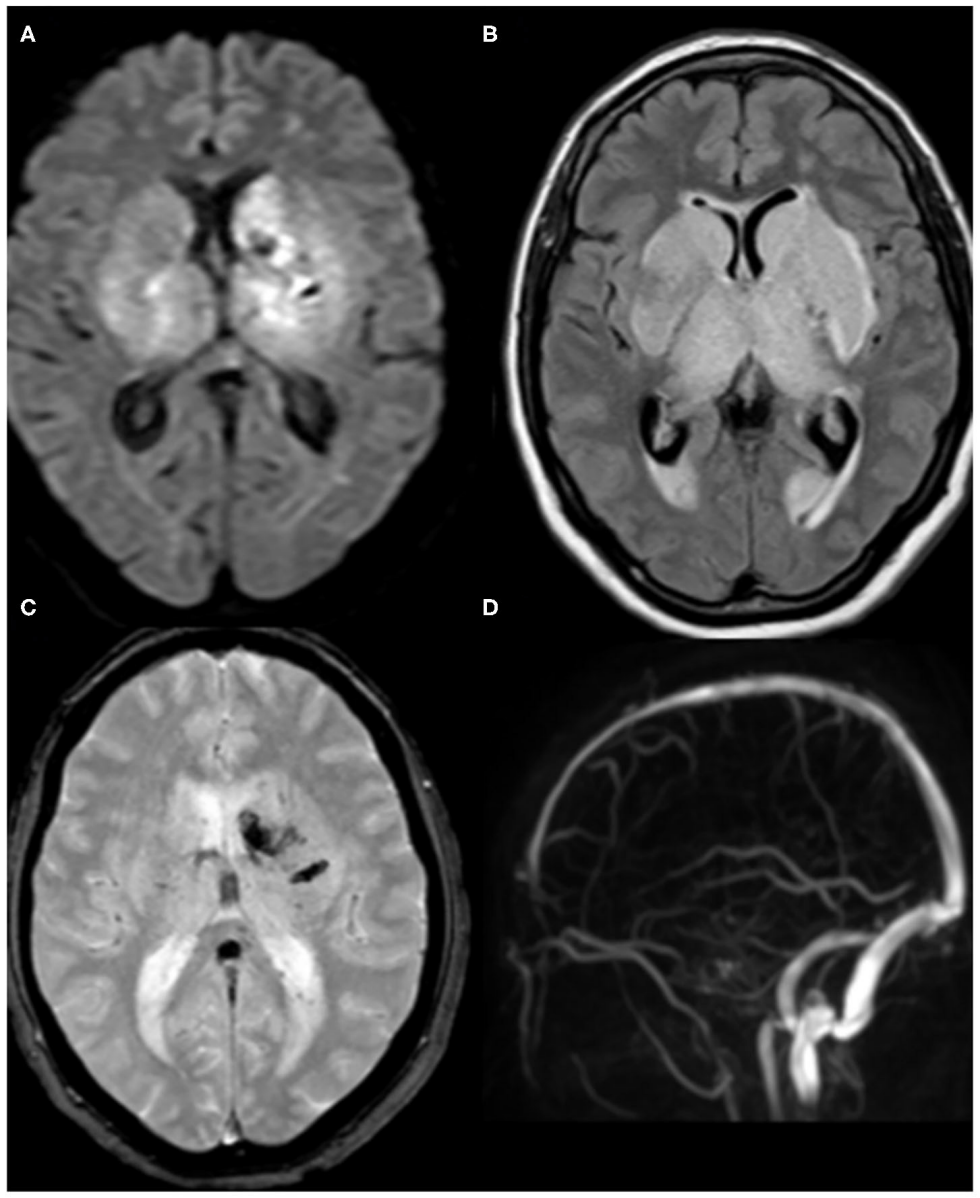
Brain MRI of a 62-year-old female patient. (A) axial DWI and (B) Fluid-attenuated inversion recovery (FLAIR) demonstrate acute infarction involving bilateral thalami and basal ganglia. (C) T2^*^-weighted imaging (T2^*^WI) shows associated hemorrhagic foci at the left side. (D) The corresponding magnetic resonance venography (MRV) reveals the absence of the normal flow in the deep cerebral veins. Findings are consistent with venous infarction secondary to deep cerebral venous thrombosis.
